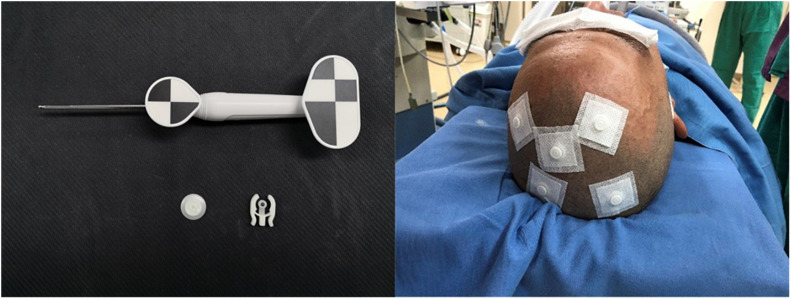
Videometric-tracked marker: it was capable of automatic patient-to-image registration, was adhered to the scalp in the preoperative and accompanied the patient for a CT scan; detected optical frame marker during surgery.
